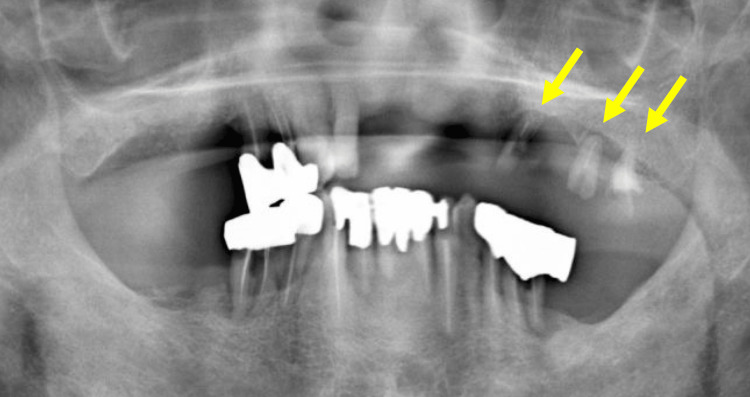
Panoramic radiograph taken at the first visit The panoramic radiograph revealed that teeth #23, #25, and #26 were residual roots. The surrounding alveolar bone was absorbed (arrow).
